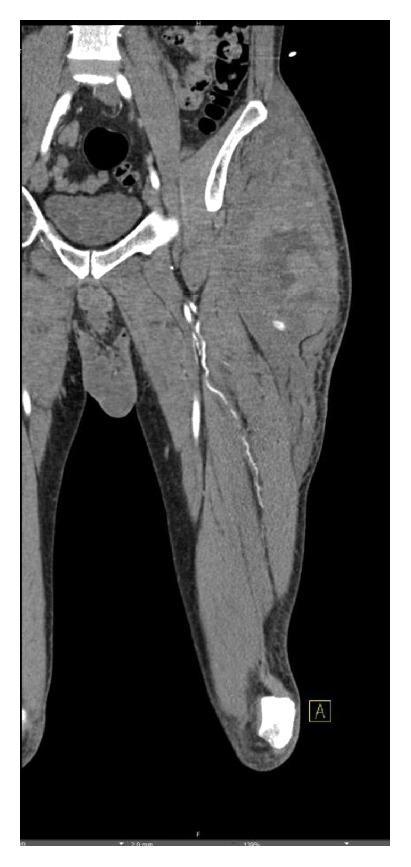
Coronal CT reconstruction further details the area of extravasation and associated hematoma.
